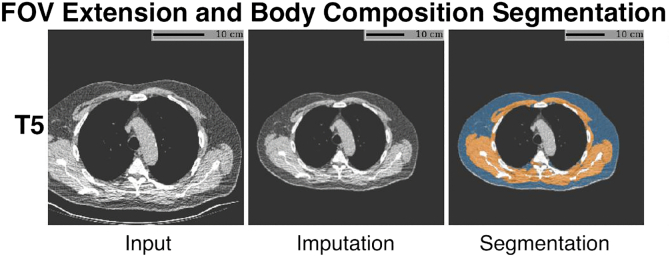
Segmentation process example. The input image shows the initial CT image. In this image part of the subcutaneous fat and part of the muscle is missing on the right side of the body. The imputation image shows the newly generated ‘complete’ image data. The segmentation image shows the segmentation generated based on the Imputation image. Orange = Skeletal muscle. Blue = Subcutaneous adipose tissue. For the skeletal muscle both area and density is computed.
